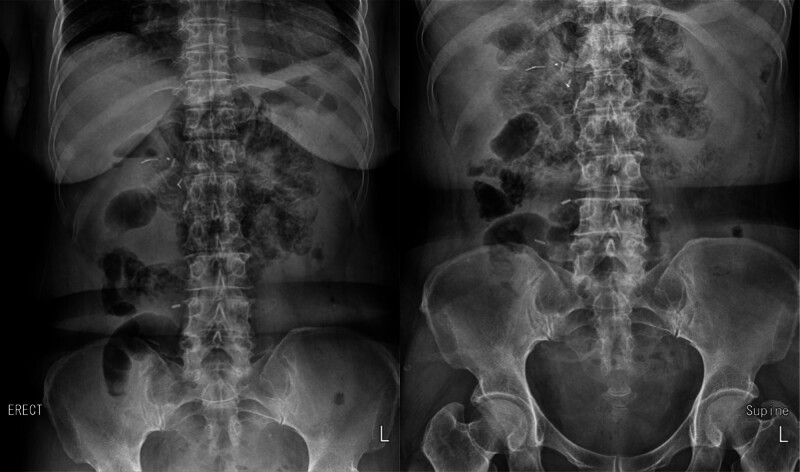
Plain X-ray of the abdomen (erect and supine) performed on the first day of hospitalization.
